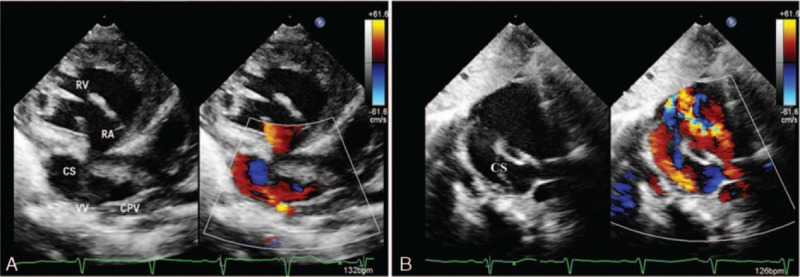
Echocardiography misdiagnosed the drainage pathway of a 3-month-old boy. (A) The irregular apical 4-chamber view shows the pathway of common pulmonary vein–vertical vein (VV)–coronary sinus (CS), the VV was identified as a narrowed left pulmonary vein by surgery. (B) The subcostal view showed a flow to CS.
